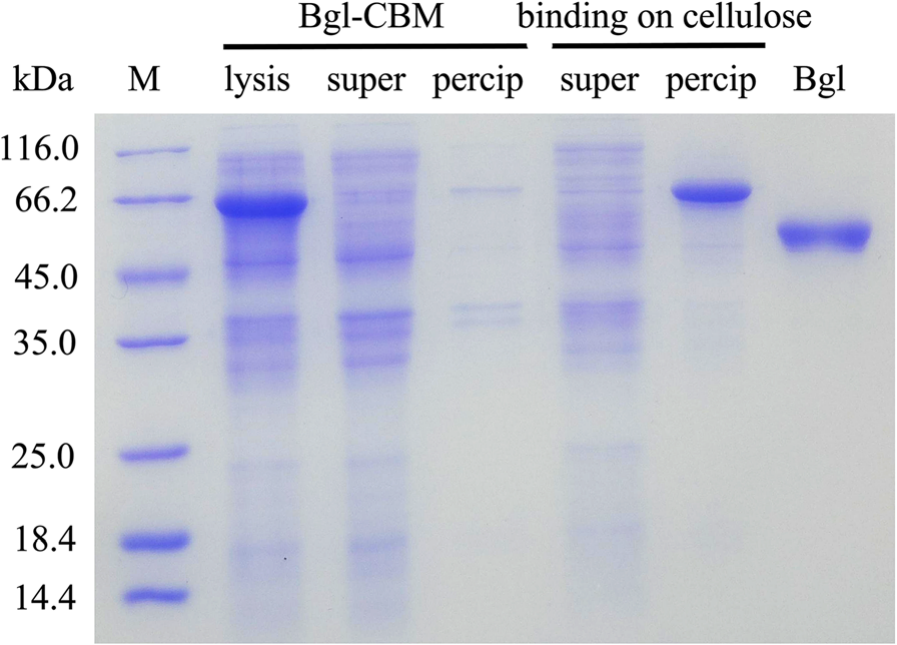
SDS-PAGE of Bgl1A(A24S/F297Y)-CBM expression in *E. coli* using light induction and adsorption onto cellulose after autolysis. M is molecular marker, lysis is supernatant of fermentation culture after cell autolysis, super and precip are supernatant and precipitate after ultrasonic fragmentation of cells that were not autolyzed. Binding on cellulose was allowed for one hour, the supernatant and precipitate were separated by centrifugation. Bgl is purified protein using hexahistidine affinity tag
